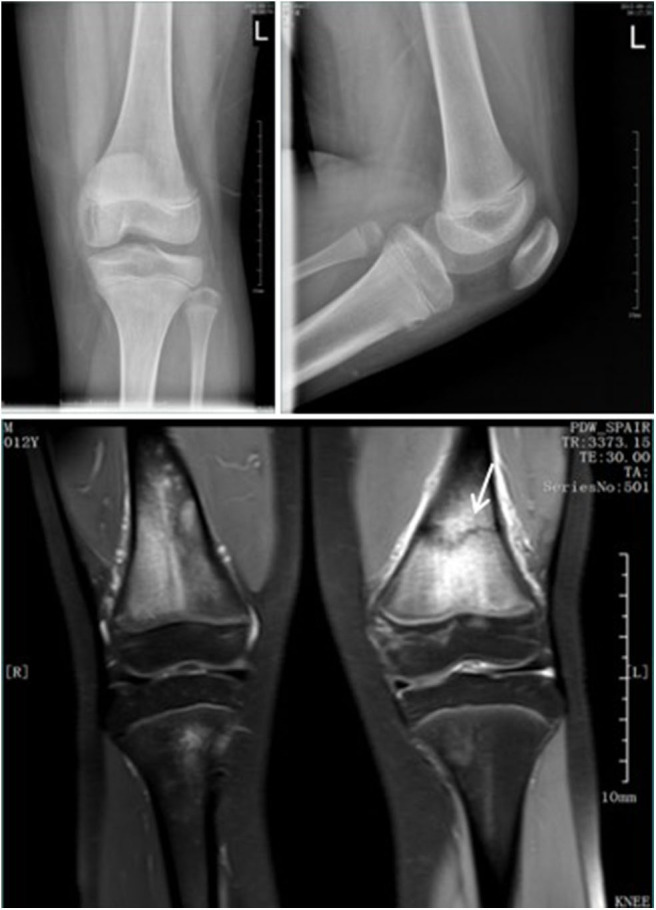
Standard radiographs in AP and LL projections did not show a fracture. MRI demontrated a fracture in the distal femur (arrow).
